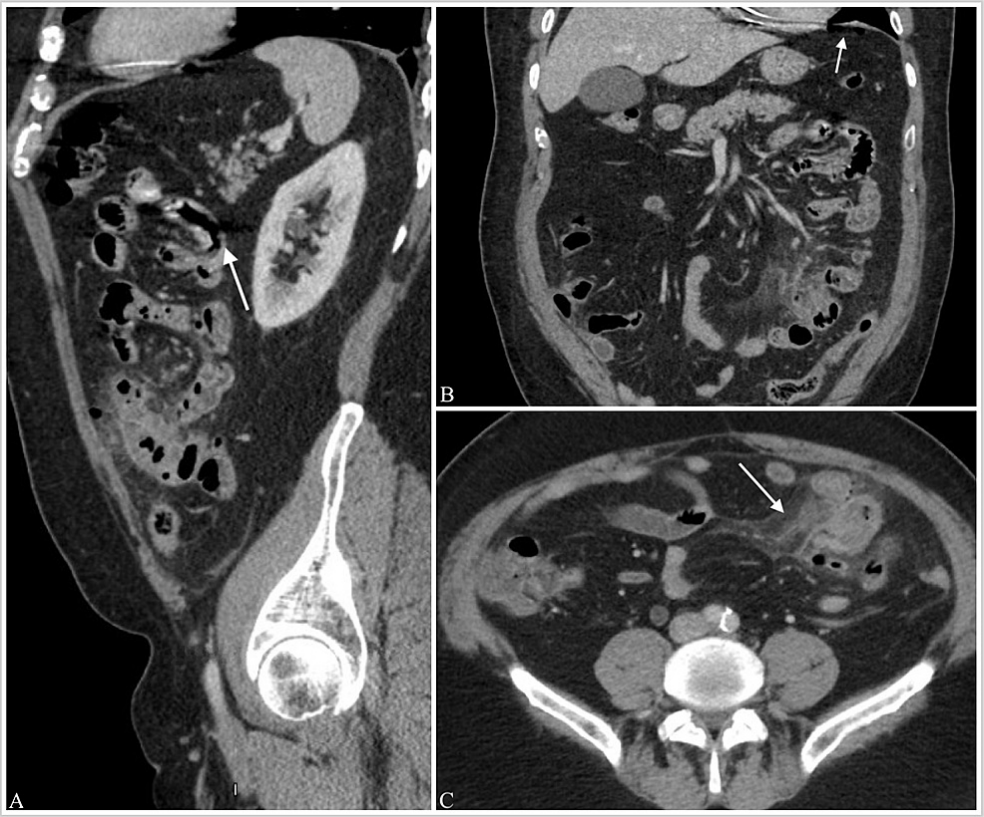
IV contrast CT scans showing (A) area of perforation, (B) sub-diaphragmatic free air, (C) pericolic fat stranding with inflammation and edema
